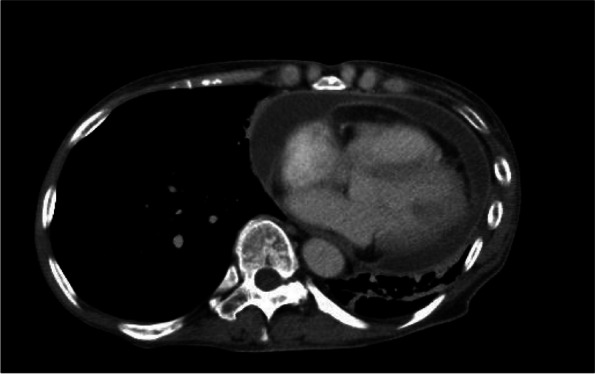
Chest CT on admission. Chest CT showed massive pericardial effusion with thickening of the pericardium
